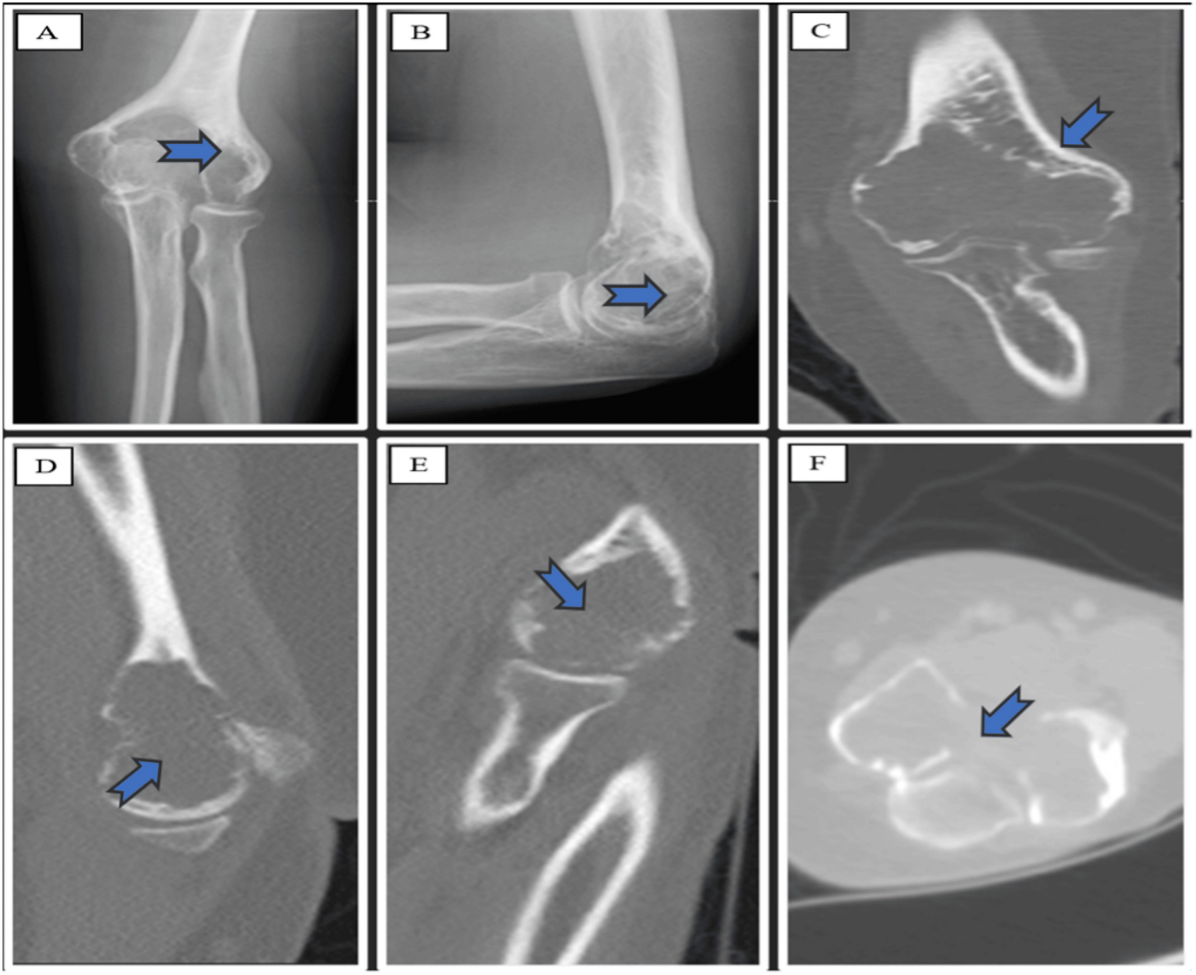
X-ray imaging and computed tomography (CT) of the left elbow revealed an epiphyseal expansile lytic lesion in the distal humerus. The X-ray and CT images of the left elbow provide a comprehensive view of the joint from various angles. The X-ray anterior-posterior (A) and lateral (B) views offer an initial assessment of the bony structures and alignment. The CT scan further enhances the visualization of the elbow in greater detail. The coronal view (C), sagittal views (D and E), and axial view (F) show a large, mildly expansile, lytic lesion noted at the distal humeral condyle abutting the articular surface.
